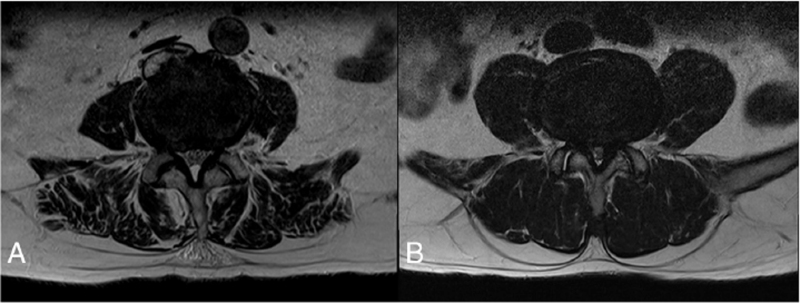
Grading system for lumbar central spinal stenosis based on T_2_-weighted axial magnetic resonance imaging findings of the lumbar spine. Lumbar central canal stenosis is present when the anterior cerebrospinal fluid space is obliterated and divided; moderate stenosis refers to the aggregation of some cauda equina, (a); severe stenosis refers to a state whereby the entire cauda equina appears as a bundle (b).
